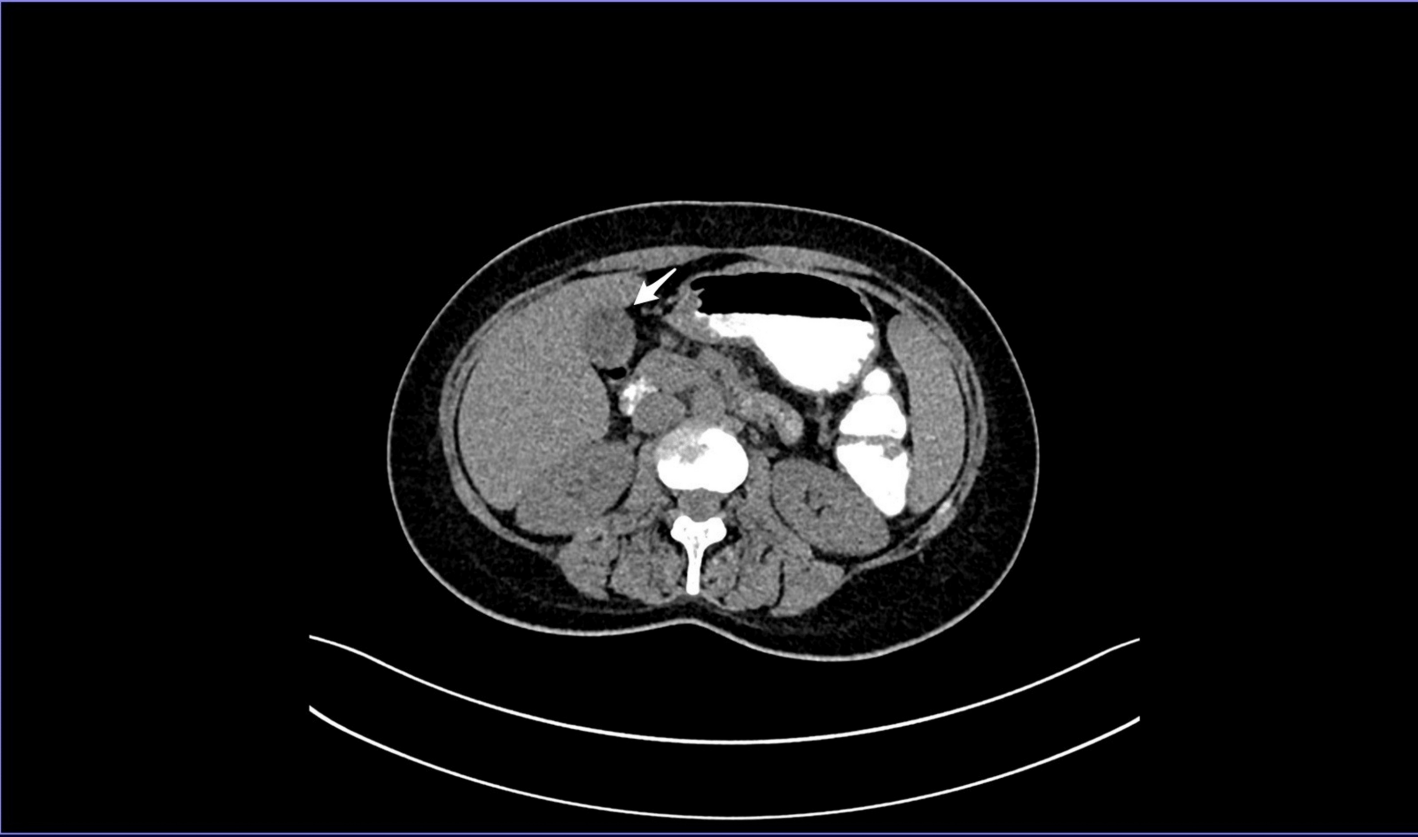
Computed tomography (CT) of the abdomen Contrast-enhanced axial CT abdomen demonstrating gallbladder wall thickening and pericholecystic inflammatory changes (white arrow) without evidence of gallstones, suggestive of acute acalculous cholecystitis.
